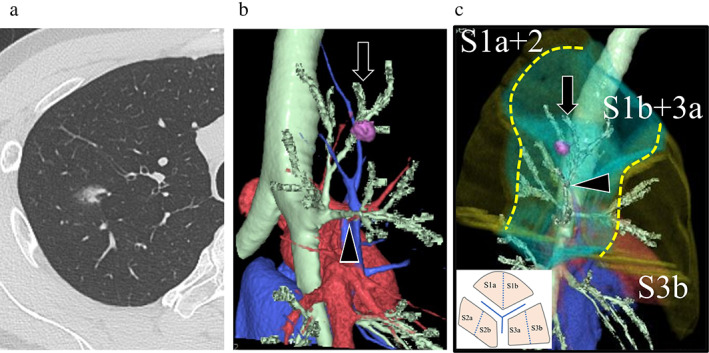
(a) Computed tomography (CT) reveals a lung tumor in the central area of the upper lobe. The tumor distance from the visceral pleura was approximately 3 cm. (b) The tumor is mainly located between B1b and B3a. B1b (arrow) and B3a (arrowhead). (c) We planned combined S1b + 3a segmentectomy using three‐dimensional CT to keep sufficient margins. If sufficient parenchyma is to be preserved, S1bii + S3ai combined segmentectomy should be selected; however, the surgery is very difficult to perform. B1bii (arrow) and B3ai (arrowhead)
